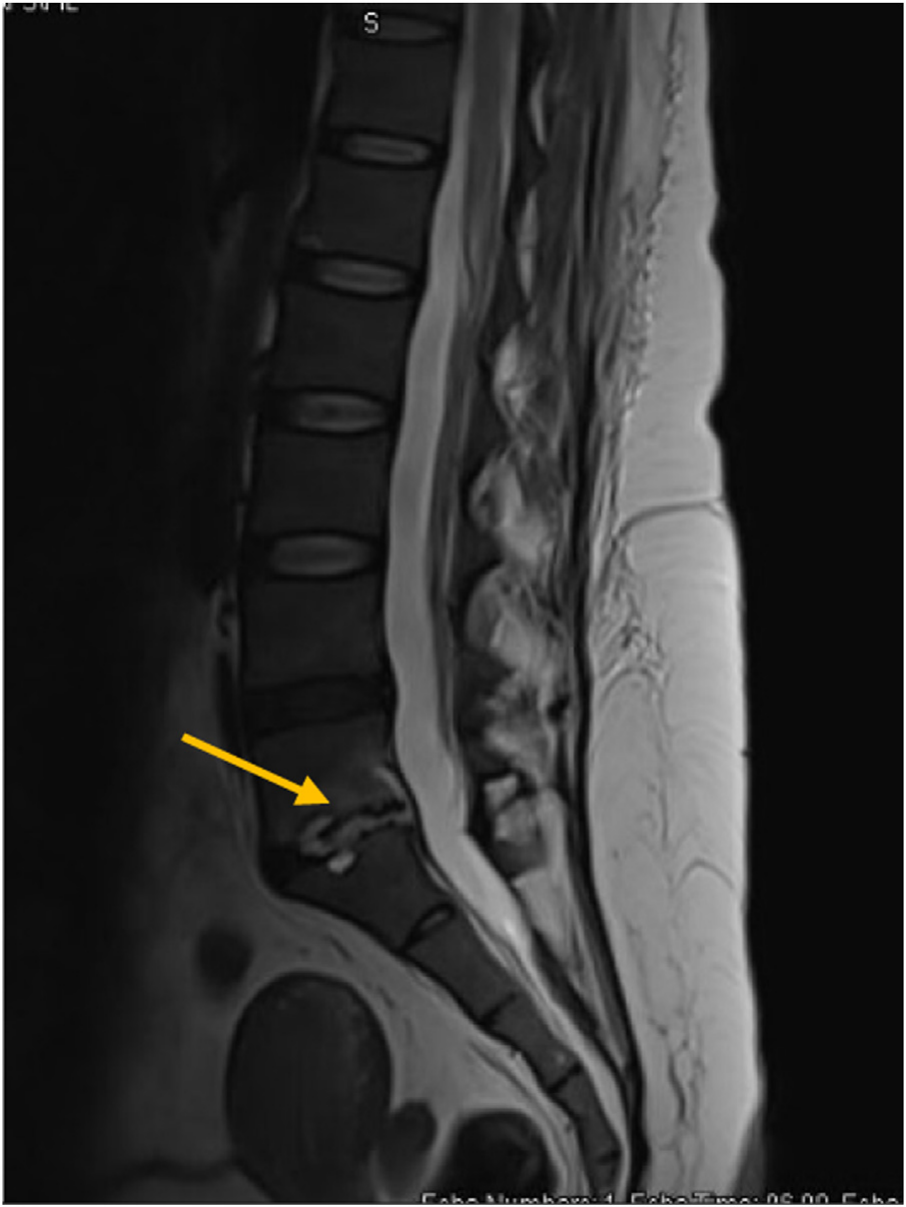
Repeated Lumbosacral MRI showing L5-S1 spondylodiscitis.
